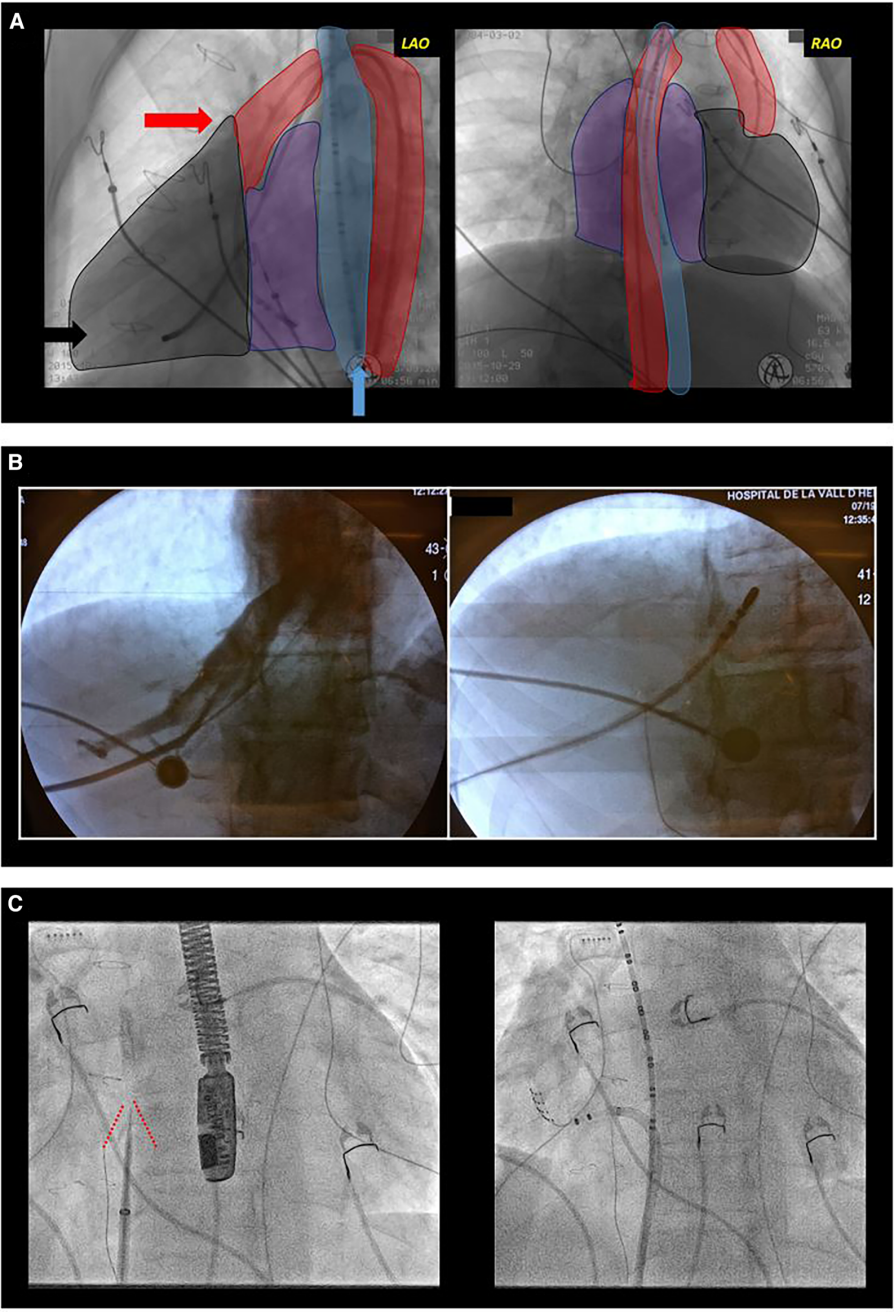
Difficult access. (A) Complex congenital heart disease with a single ventricle type, mesocardia, and dextroapex, initially repaired with classic Fontan surgery which was later converted to Fontan with intracardiac tube. Electrophysiological study was performed due to syncopal supraventricular tachycardia. Conventional radiological projections are shown: left anterior oblique (LAO, left image) and right anterior oblique (RAO, right image). The outlines of the esophagus (blue), aorta (red), ventricle (black), and atria (purple) are shaded. Due to the absence of femoral venous access, a transesophageal diagnostic catheter (blue arrow) was introduced for atrial sensing, and through the femoral artery, a tetrapolar catheter to the ventricle (black arrow) for rescue ventricular stimulation and a mapping-ablation catheter for treatment (red arrow) were progressed. (B) Transhepatic access guided by fluoroscopy. In the first fluoroscopy image, contrast injection into the hepatic venous system can be observed. In the second image, the catheter is progressed through this venous system. (C) Transeptal access in a patient with transposition of the great arteries corrected with atrial switch surgery (Senning). Both fluoroscopy images show an left anterior oblique projection. In the left image, a transeptal puncture needle oriented anteriorly and towards the right shoulder. Tenting of the interatrial baffle is observed (red lines). In the right image, a mapping-ablation catheter has been advanced through a long sheath to the neo-pulmonary venous atria.
